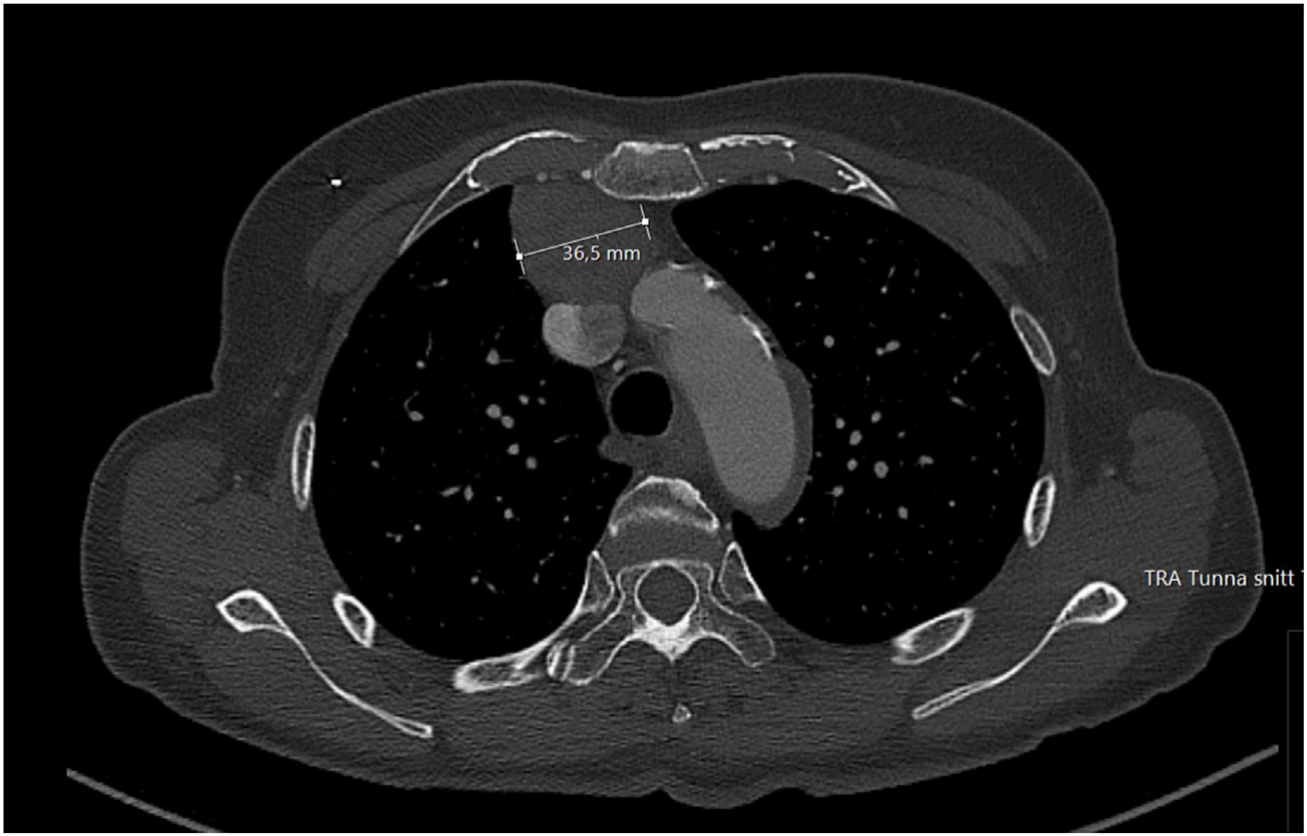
Computed tomography demonstrating mediastinal tumour.
